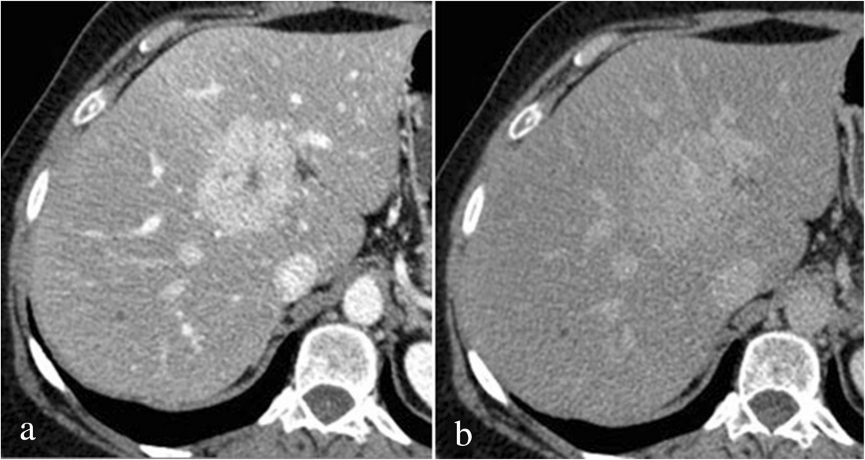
Single-pass 64-detector row computed tomography findings of focal nodular hyperplasia in 53-year-old woman. Mixed phase (hepatic arterial phase/portal venous phase during hepatic enhancement) shows intense homogeneous enhancement with hypodense focal central scar (a); on delayed phase (b) the lesion appears substantially isodense to liver parenchyma with persistent enhancement of central scar.
